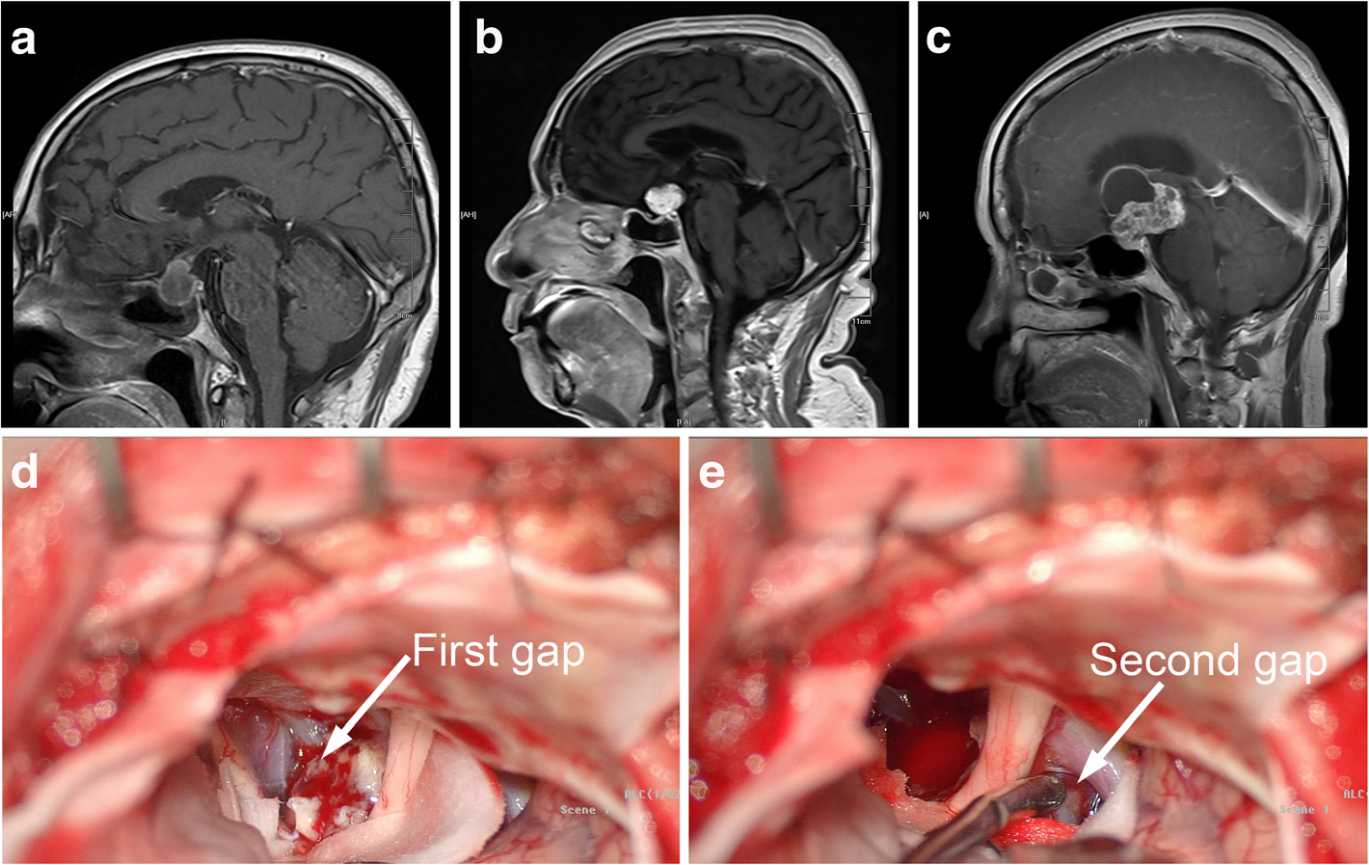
Three common craniopharyngioma MRI’s sagittal and two gaps of tumor exposure under the supraorbital visual field. a intra-suprasellar, b suprasellar-extrayentricular, c intra-suprasellar-intraventricular, d expose tumor from the first gap, e expose tumor from the second gap
